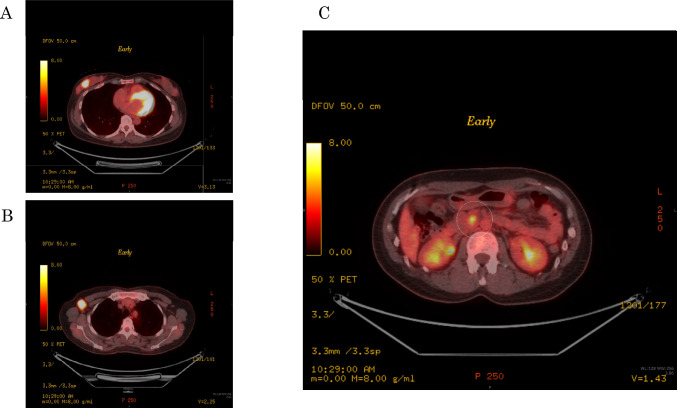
FDG-PET/CT. Accumulation is noted in the right breast (A), axillary lymph nodes (B) and pancreas head (C, circle)
